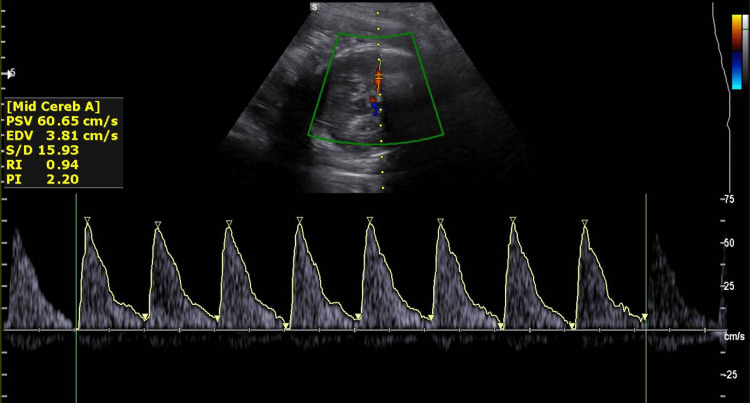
Doppler velocimetry with elevated middle cerebral artery peak systolic velocity indicative of fetal anemia. PSV: peak systolic velocity, EDV: end-diastolic volume, S/D: systolic/diastolic ratio, RI: resistive index, PI: pulsatility index.
